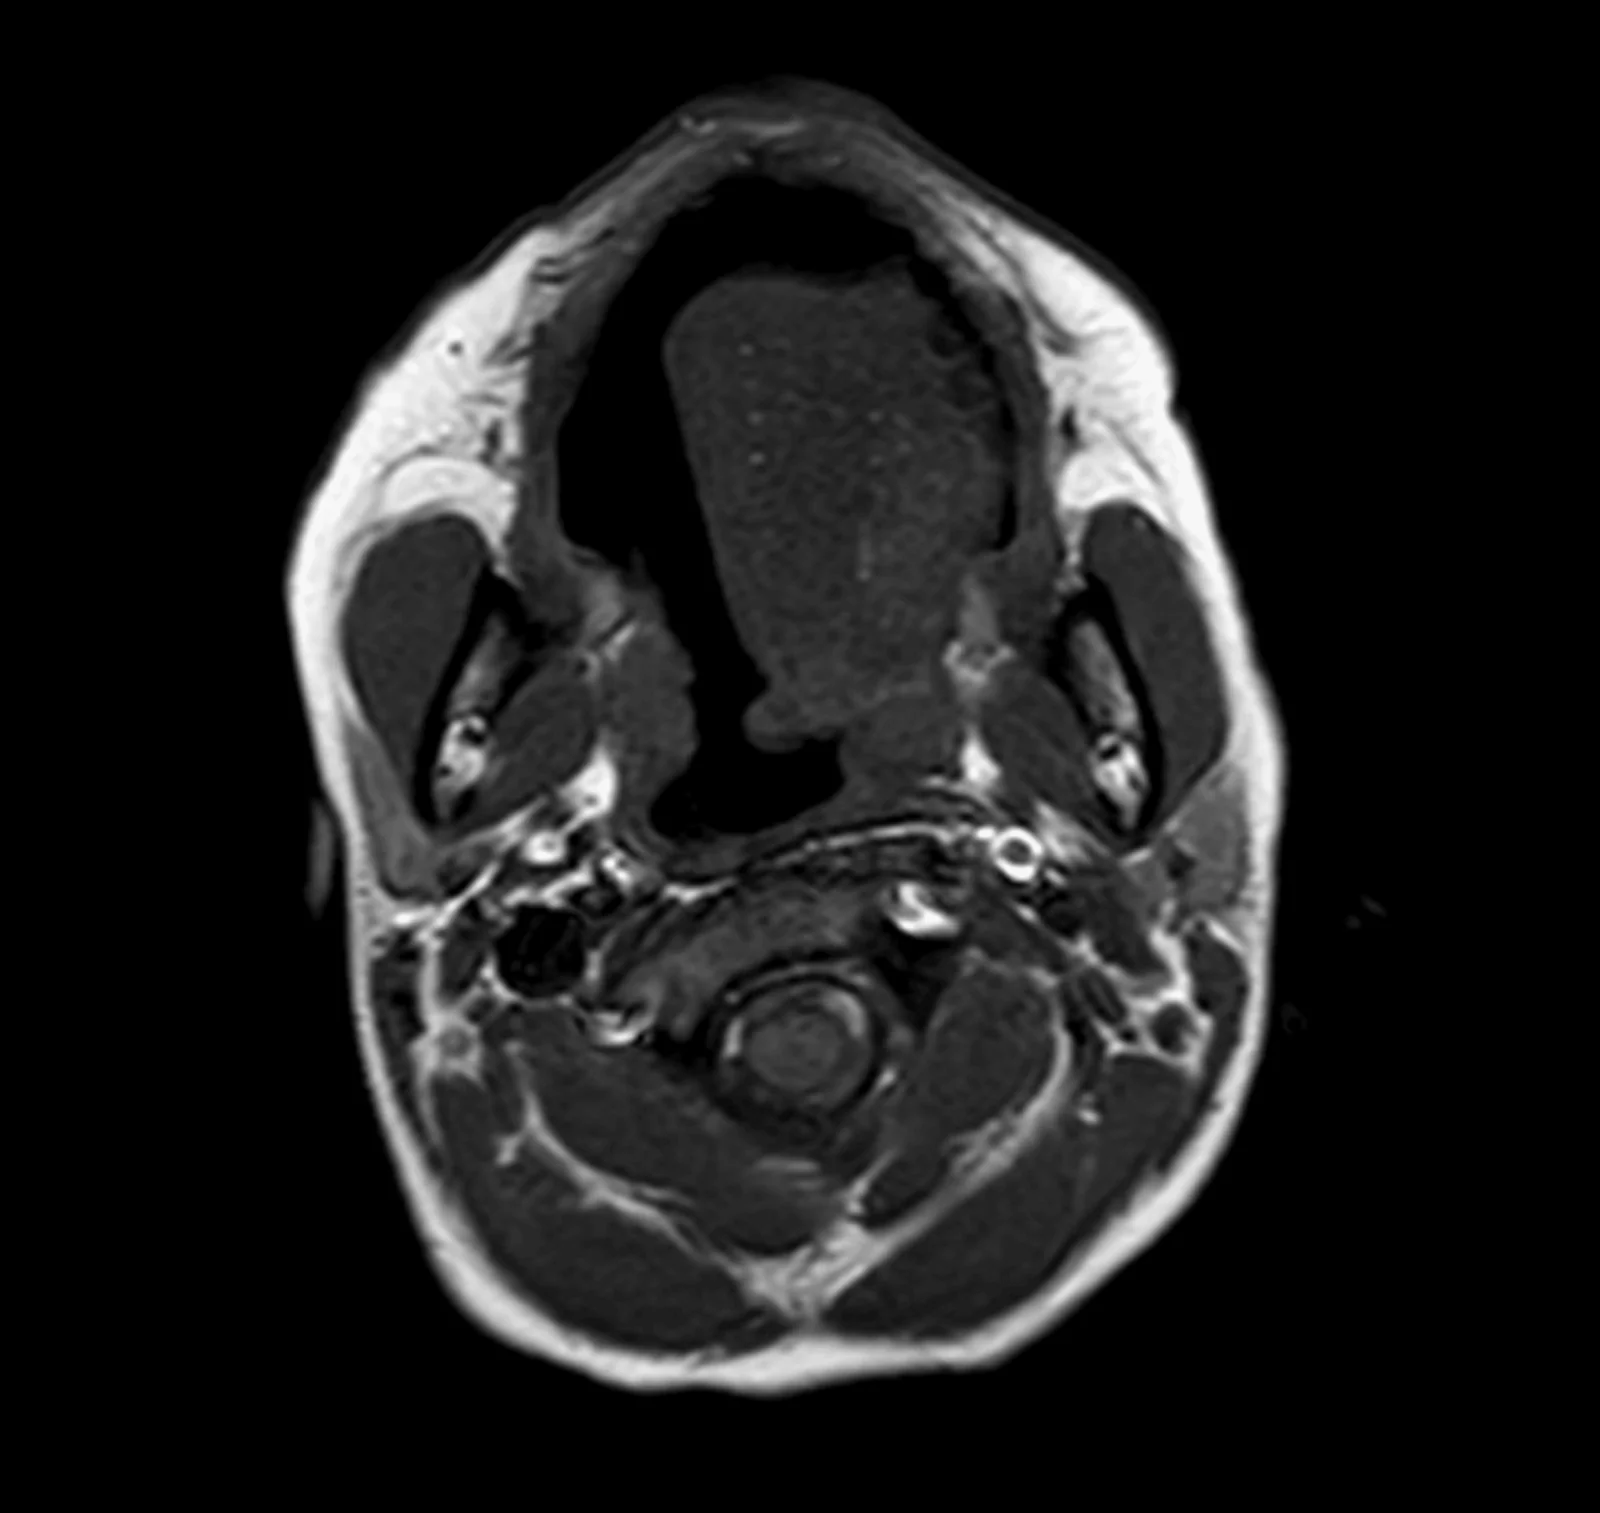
Axial T2-weighted image at the level of the posterior fossa. Normal signal intensity of the cerebellum and brainstem. Patent fourth ventricle and no evidence of obstructive hydrocephalus.
